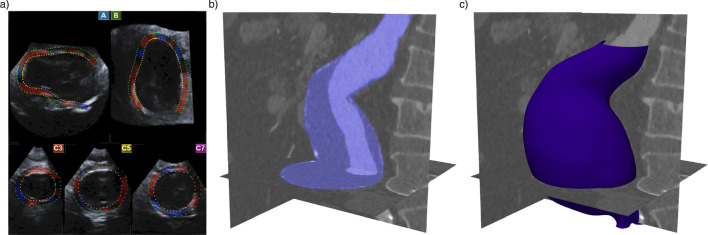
AAA of patient 3 with a maximum diameter of 64.0 mm. a Shows five 2D cross section of a volumetric ultrasound data set, two longitudinal cross sections in the sagittal (A) and frontal (B) plane and three transversal cross sections (C3, C5, and C7) at different positions along the AAA. Segmentation of the vessel wall area as region of interest for the wall motion tracking was performed manually in the A and B planes and then automatically completed by the algorithm in the whole image volume. The false masking inside the vessel is called pseudo-apex and will be removed in the further model setup. b The same AAA as in image a) based on CT-A imaging. The complete AAA is highlighted. The darker areas are the ILT, the light area is the blood perfused lumen with contrast agent, and the bright shining areas around the vessel wall are calcifications. c Same image as in b) with the reconstructed vessel wall as surface plot after smoothing
